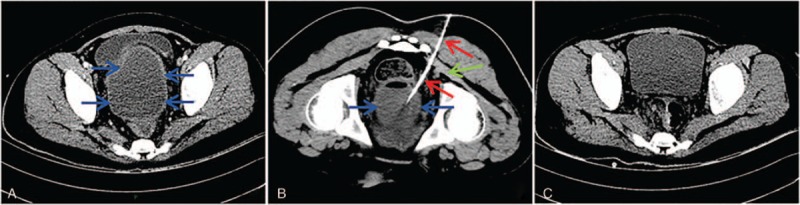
Transgluteal approach. (A) Axial CT scans revealed that 8 × 9 cm abscess (blue arrow) is detected in the excavation rectovesicalis. (B) Axial CT scans (prone position) showed a 17-gage coaxial needle (red arrow) advanced progressively into the abscess cavity (blue arrow) through the piriformis (green arrow). (C) Axial CT scans obtained 2 weeks after the removal of drainage catheter revealed that abscess cavity completely collapsed.
